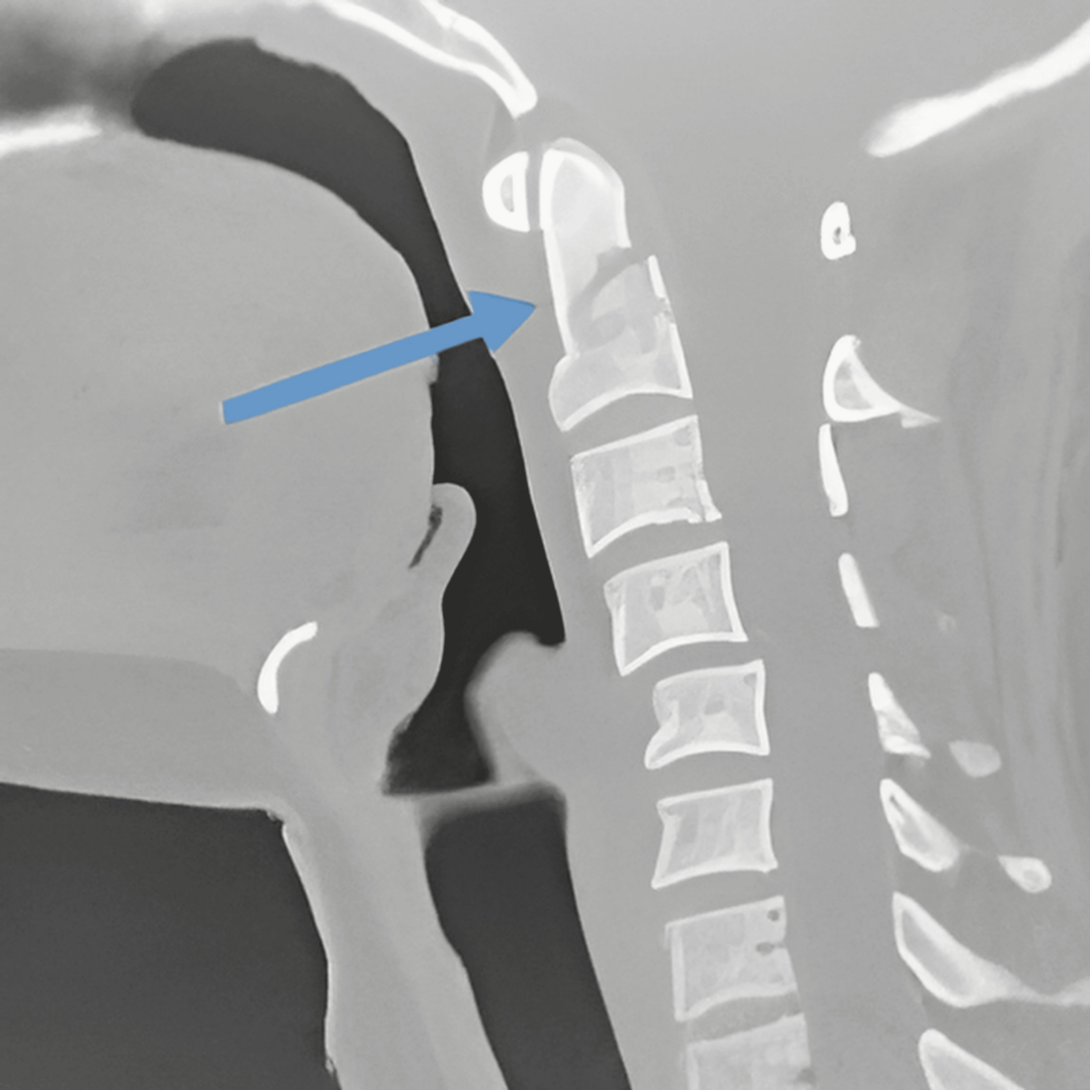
The CT scan shows a type II odontoid fracture in the first case (blue arrow).
